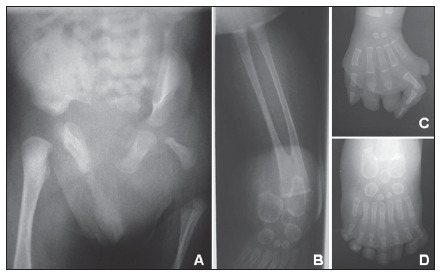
Radiographs showing hypoplasia of the left iliac bone and femur (A), left leg with absence of tibia and duplicated fibula (B), left hand with absence of distal phalanges of the second, third and fifth fingers (C) and mirror polydactyly of the foot (D).
